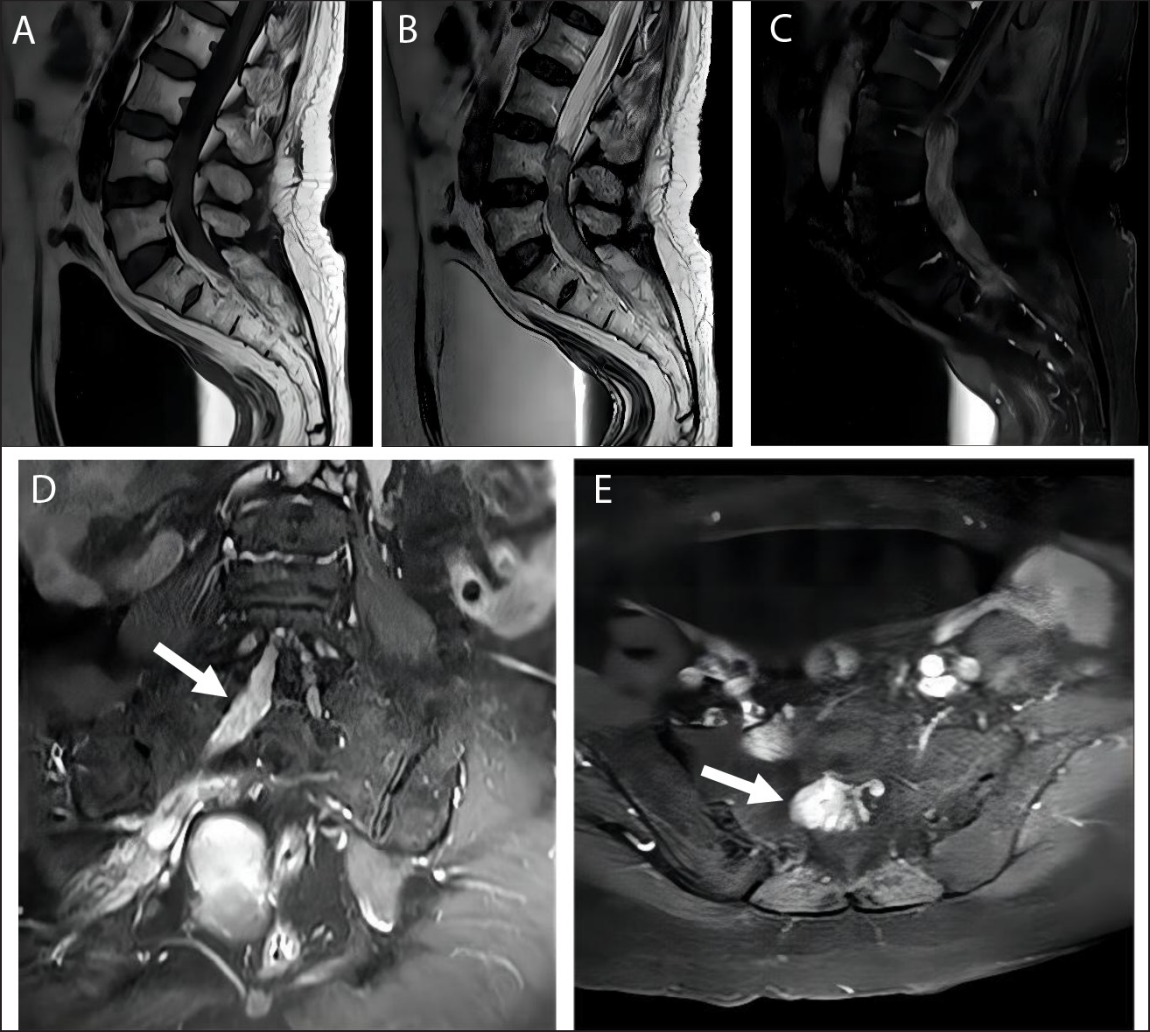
MRI images of SS in spinal canal. T1WI sagittal (1A) displays a striped, iso-signal mass within the spinal canal; T2WI sagittal view (1B) reveal an iso-signal mass with mixed patchy high signal areas; T1WI enhanced sagittal view (1C) shows significant inhomogeneous enhancement; T1WI coronal view (1D) and axial view (1E) show (arrow) demonstrate that part of the lesion extends outward along the first and second sacral foramen on the right.
